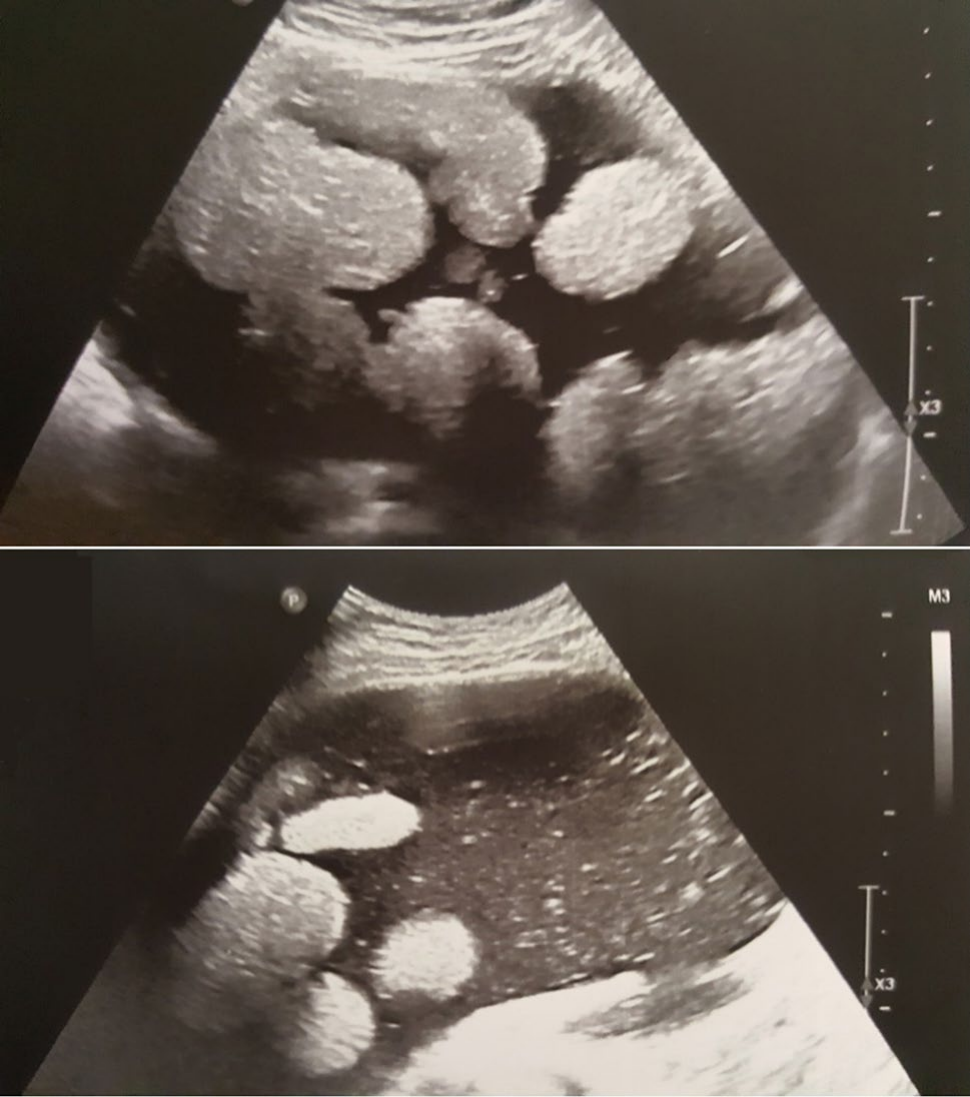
Ultrasound examination allows visualization of the movement of the balls inside the tumor cyst
